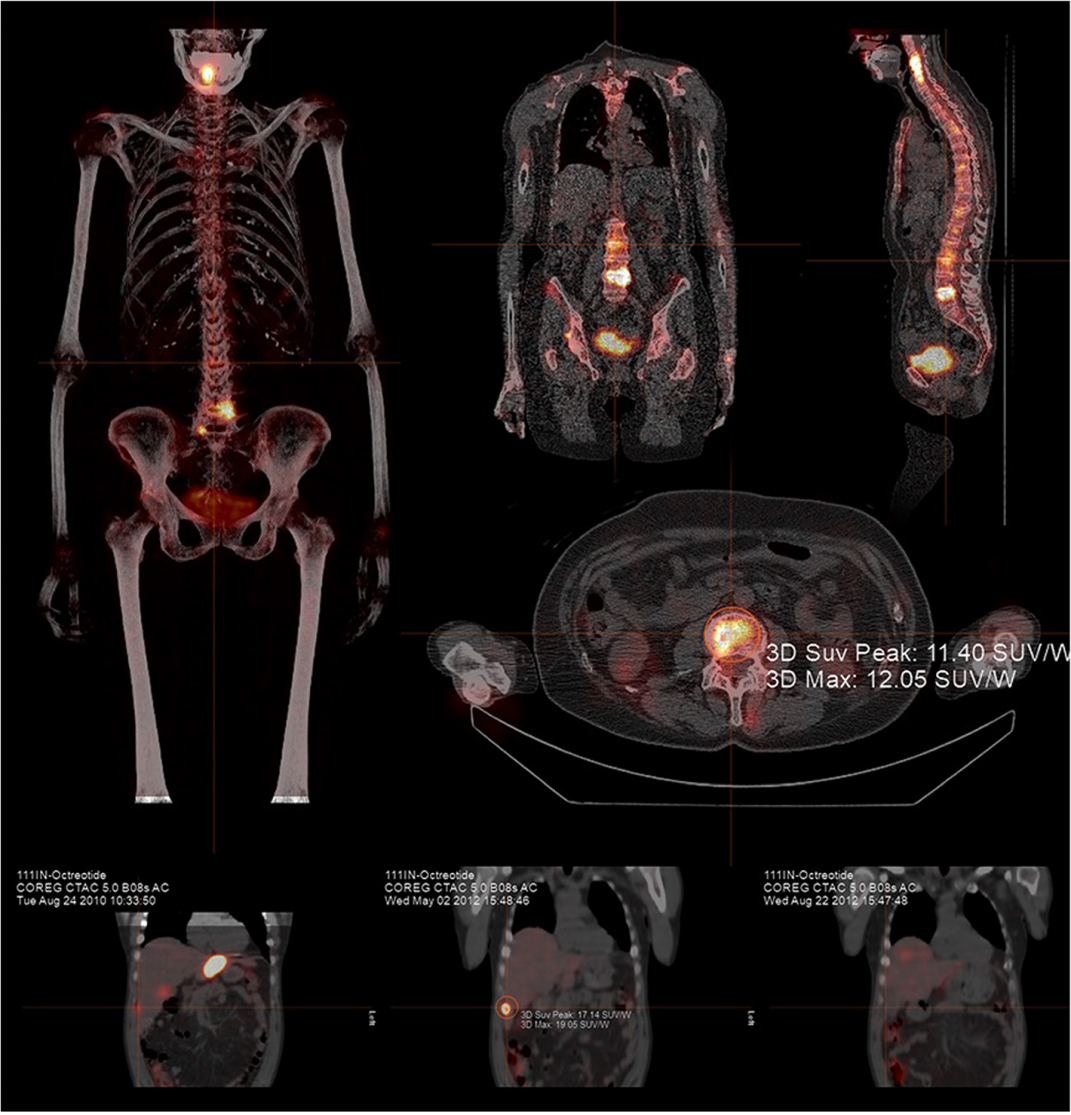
Absolute quantification is possible with Hybrid Recon™ (Hermes Medical Solutions). An SUV_max_ of 12.05 and an SUV_peak_ of 11.40 were generated in the delineated area of this patient’s lumbar spine. (Images courtesy of Hermes Medical Solutions, London, UK)
